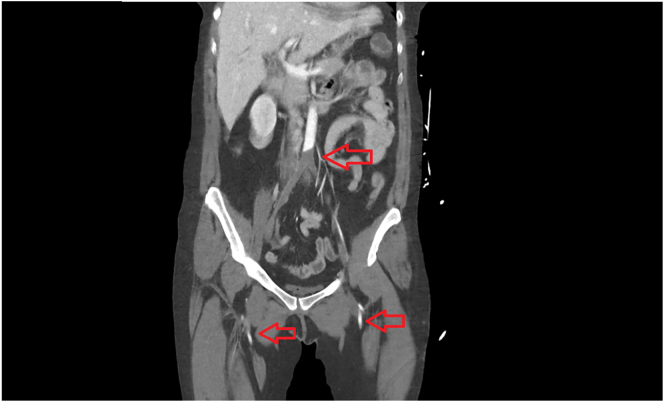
Coronal computed tomography angiography showing aortoiliac occlusion. Reconstitution of flow is evident in the bilateral superficial femoral arteries.
